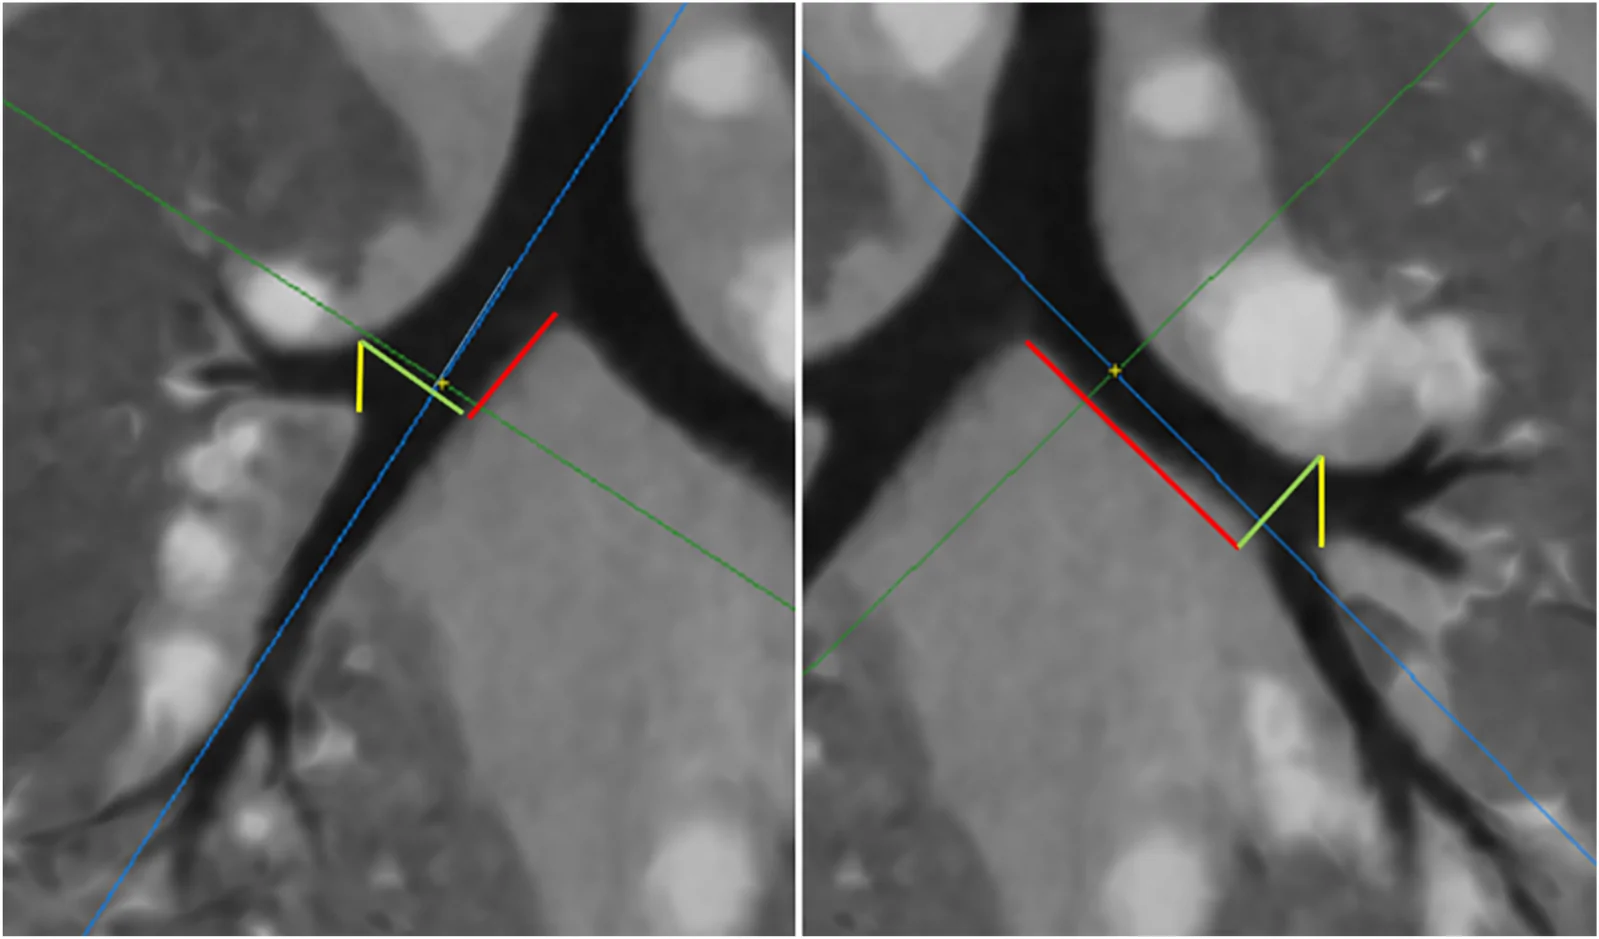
Computed tomograms showing how the bronchial lengths are measured after each bronchus is reconstructed along its long axis. Step 1. Draw a line (yellow) across the upper lobar bronchus from the inferior point of origin. Step 2. Draw a line (green) across the lower end of the main bronchus from the upper tip of the yellow line. Step 3. Measure the length (red line) from the inferior tip of the green line to the carinal bifurcation point.•BLR: ratio of the longer to shorter main bronchus•Aortic arch position relative to the trachea: left, right, or double.
